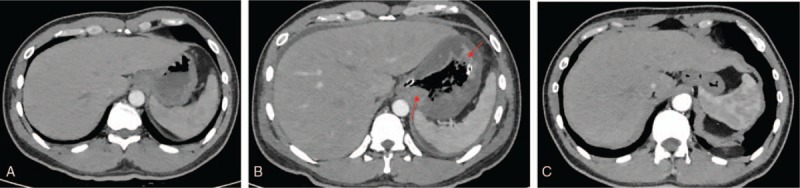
(A) Enhanced computed tomography (CT) scan on admission. No abnormalities were found. (B) Enhanced CT scan the 2nd day after phlegmonous gastritis. There is diffused mural thickening of the stomach with hypodense areas within the wall (arrows). (C) Gastric thickening improved remarkedly more than 2 months after phlegmonous gastritis.
